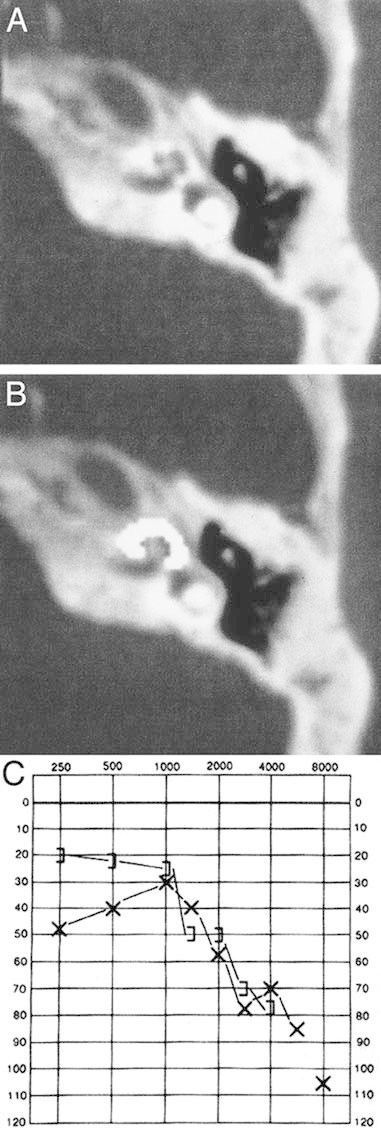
(A ) Example of a planar quantitative computed tomography image from a 66-year-old subject with Paget's disease moderately involving the left temporal bone. (B ) The same image showing the cochlear capsule regions of interest defined for this temporal bone. (C ) Air and bone conduction audiogram from the same subject and ear. 19 (Monsell EM, Cody DD, Bone HG. Measurement of regional bone mineral density: a new techniqe for the evaluation of the temporal bone. *Am J Otol* 1993;14:455–459.)
